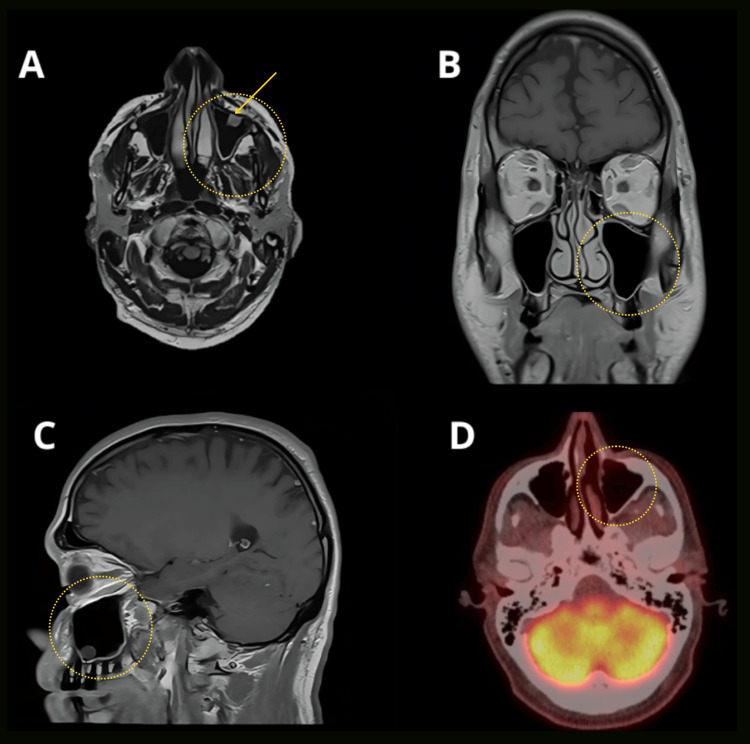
One-year post-treatment brain MRI with and without contrast, and PET/CT imaging. A. Axial T1-weighted image with contrast showing no mucosal thickening or residual lesion; a mucosal retention cyst is indicated by the arrow.
